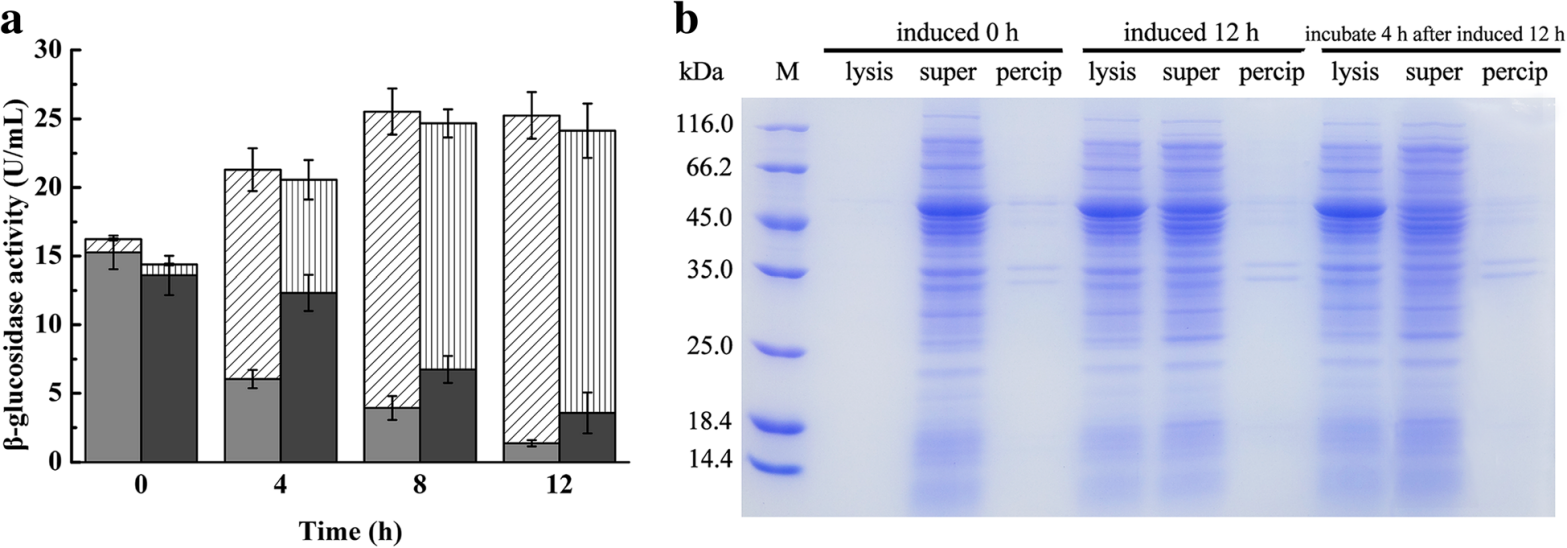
Bgl1A(A24S/F297Y) activity and SDS-PAGE assay of extracellular and intracellular fraction after cell autolysis in flasks. Slash (extracellular) and gray (intracellular) reflect β-glucosidase activity when *SRRz* lysis gene was induced with IPTG, vertical (extracellular) and dark gray (intracellular) reflect enzyme activity when autolysis was induced with lactose (a). The soluble and insoluble fraction of cell lysate was assayed by SDS-PAGE when autolysis was induced with lactose (b), M is molecular marker, lysis is supernatant of fermentation culture after cell autolysis, super and precip are supernatant and precipitate after ultrasonic fragmentation of cells that were not autolysed
